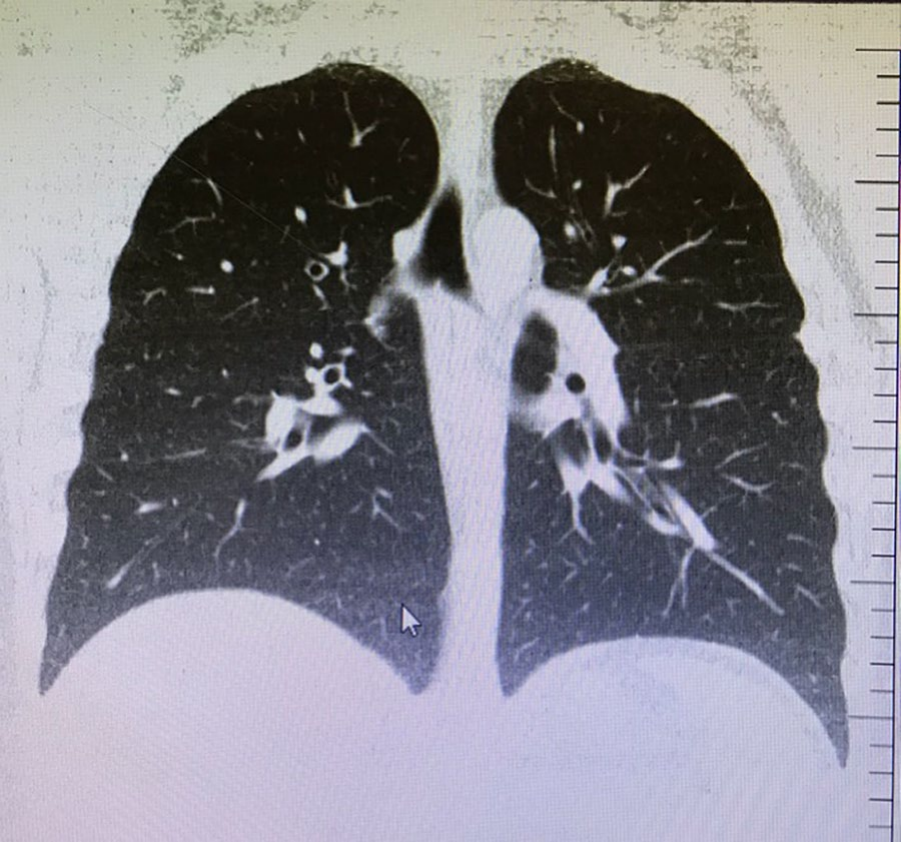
Computed tomography of the pulmonary parenchyma showing no sign of pneumonia.
